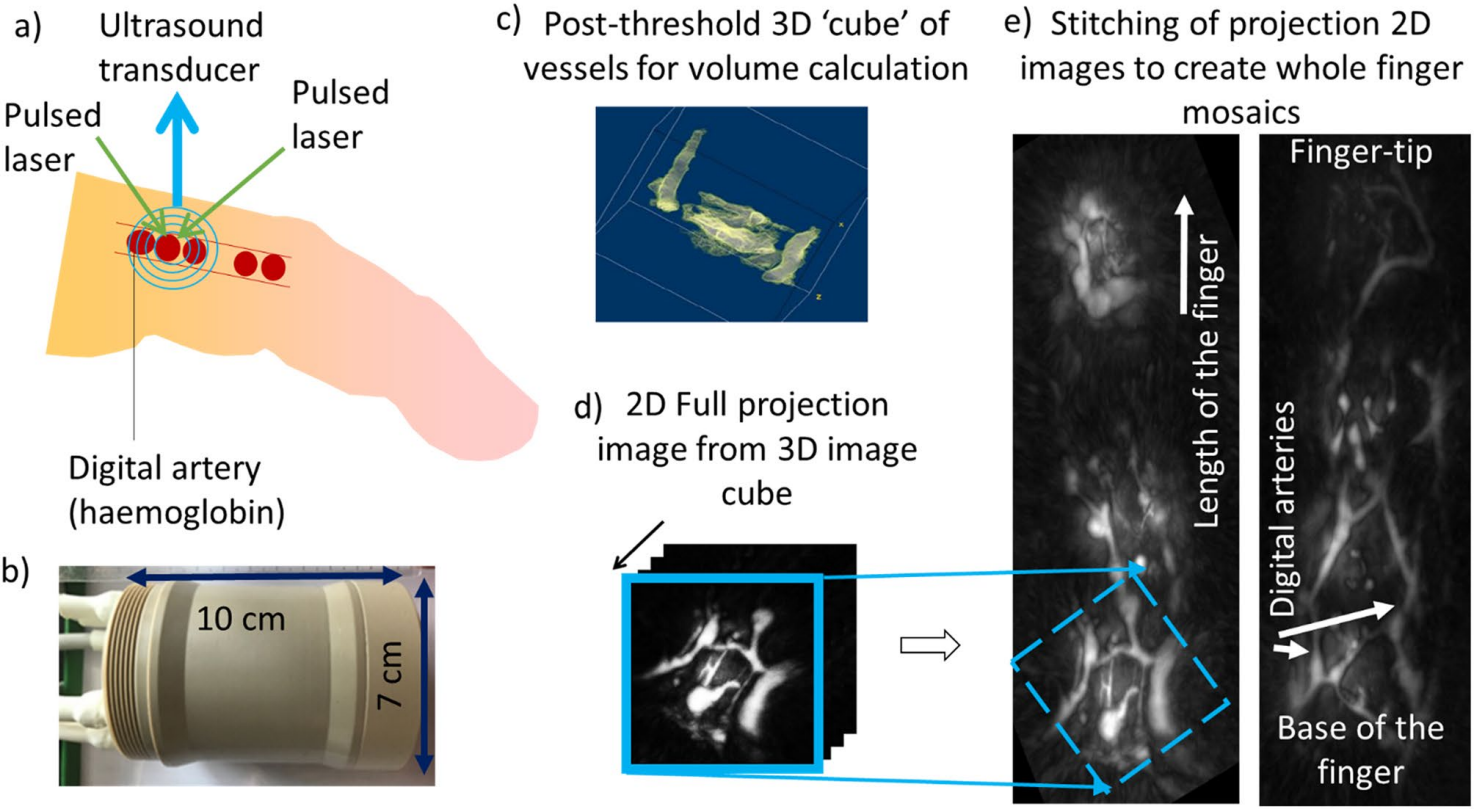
(a) Photoacoustic imaging technique. Pulsed laser light is incident on the tissue. The laser light is preferentially absorbed by the red blood cells due to the haemoglobin absorption spectrum. The light is converted to heat and the tissue generates acoustic waves detected by an ultrasonic transducer; (b) Cylindrical transducer used to collect images; (c) example of a post-threshold structural image generated in 3D (here the presence of haemoglobin, thus digital vessels, is indicated in lighter pixels); (d) representation of the creation of a projection image with frames from the cube being compressed into a 2D image. (e) examples of 2D projection full finger scans (left patient with SSc and right healthy control) indicating that digital arteries can be visualised in patients as well as controls facilitating quantitative measures of volume.
